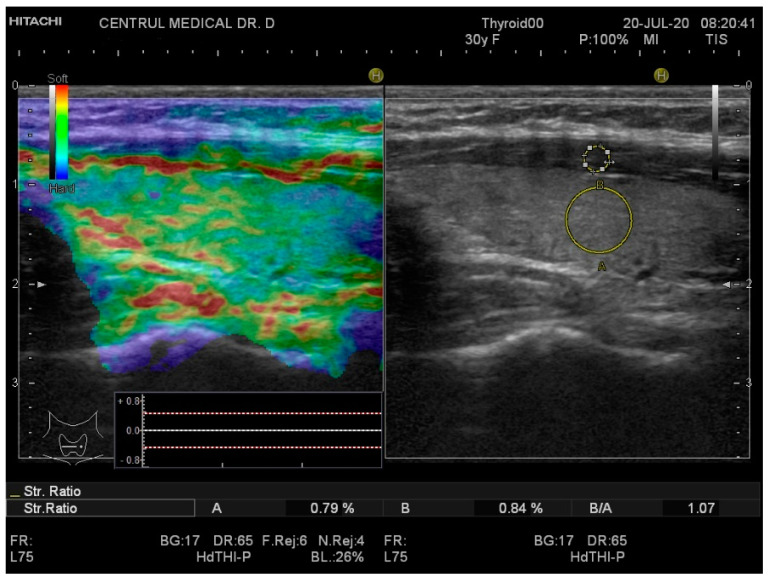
Strain elastography (left) and grey-scale ultrasound (US) (right) of a healthy patient—left thyroid lobe. The yellow circle A is the local region of interest (ROI) on the thyroid tissue; the yellow circle B is the local ROI on the sternocleidomastoid muscle adjacent to the thyroid. Strain ratio (SR) = 1.07.
